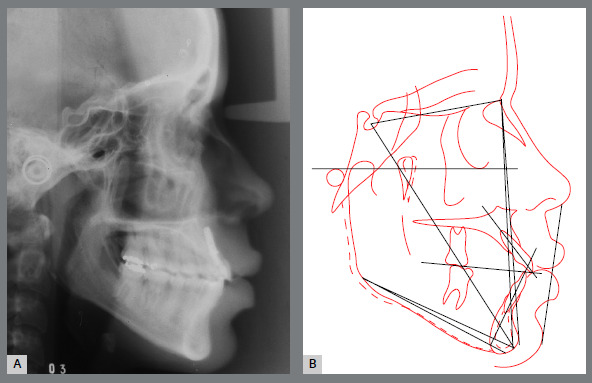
Post-treatment lateral cephalometric radiograph (A) and cephalometric tracing (B).
